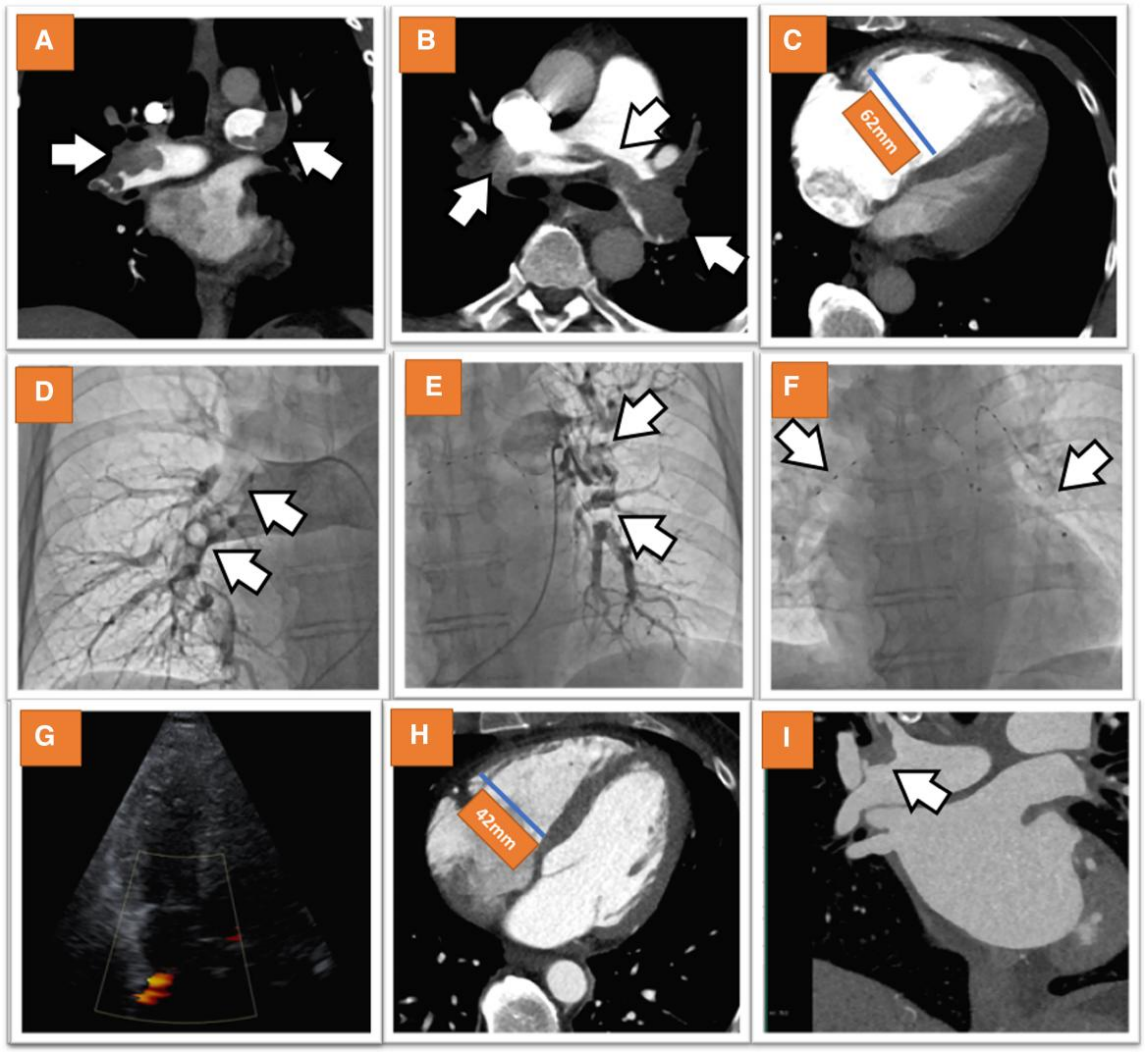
CTA showed massive, bilateral acute PE (*A* and *B*) and massive dilatation of the RV (*C*). Pulmonary angiography confirmed massive bilateral PE (*D* and *E*) and two EKOS catheters were inserted in the right and left pulmonary arteries under fluoroscopic control (*F*). Echocardiography on the next day demonstrated complete resolution of RV enlargement with normal RV diameter of 42 mm and function (*G*). Control CTPA after 2 weeks demonstrated normalization of the RV diameter (*H*) and small amounts of residual thrombi in the pulmonary arteries (*I*).
